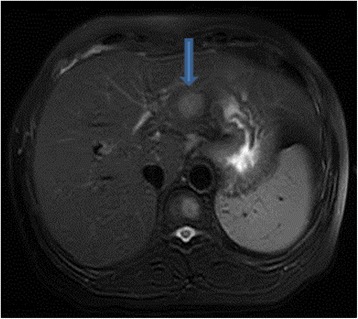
Portal phase extracellular Gd-chelate-enhanced MRI demonstrating a T1 and T2 hypointense sphenoid lesion (*arrow*) in the Sg 3 which is enhanced in the delayed phase, indicating a small hepatocellular carcinoma
